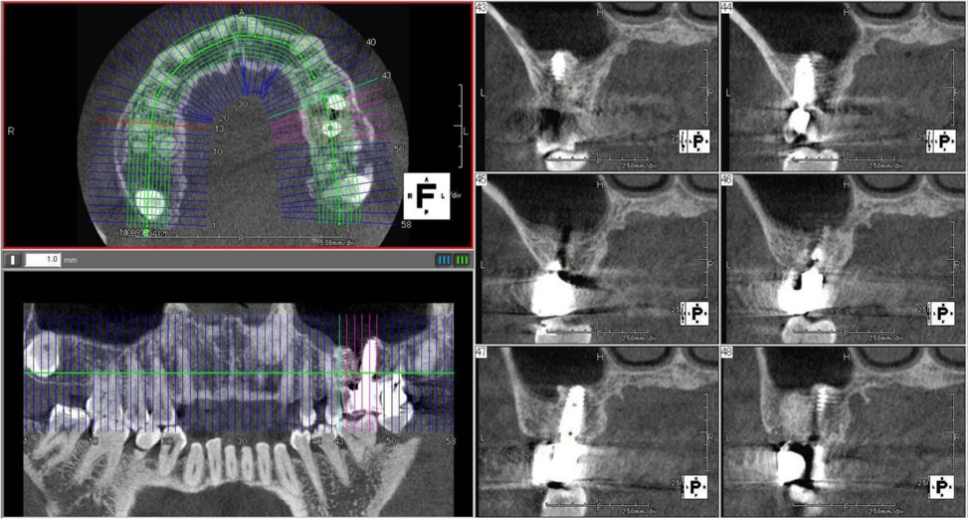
Case 1. April 2013. Cone-beam CT obtained 6 years and 5 months after superstructure placement. The double implants were surrounded by an adequate amount of hard tissue that acted as bone
